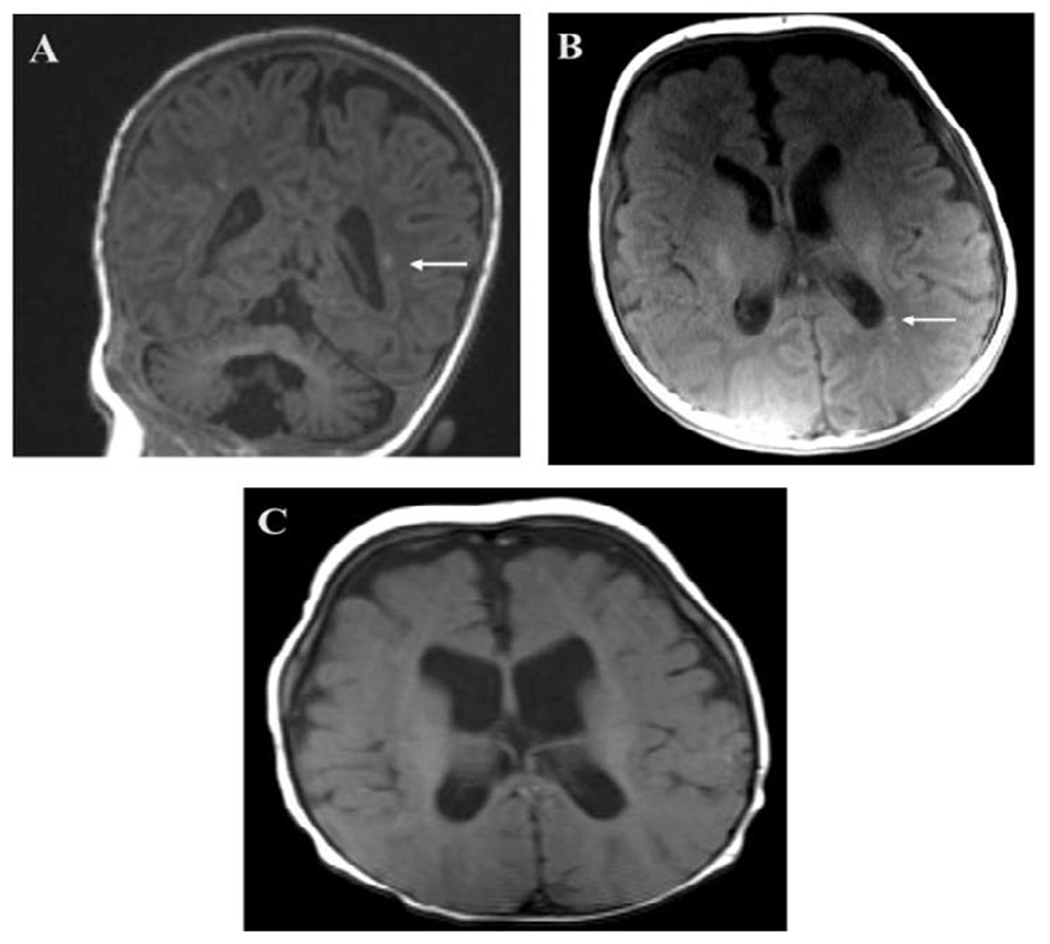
Representative brain MRI showing progressive periventricular leukomalacia (PVL). T1 images from scan at 2 months of age (A and B) showing areas of hyperintensity suggesting early PVL marked with white arrows. T1 image from follow up scan at 5 months of age showing ventricular enlargement compared to prior and ex vacuo of the periventricular white matter as evidence of late stage PVL.
